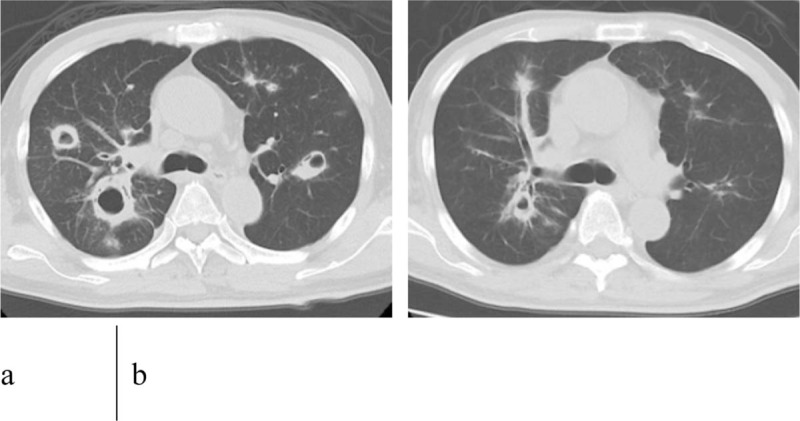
Chest CT shows changes in lung metastases. Just before initiating nivolumab treatment (A), and after 2 months of nivolumab treatment (B). CT = computed tomography.
